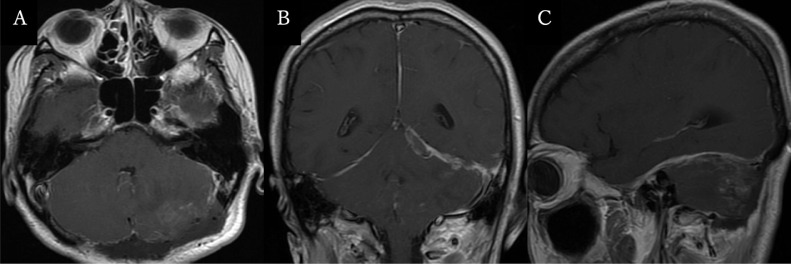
Postoperative magnetic resonance images, axial (A), coronal (B), and sagittal views (C), showing the tumor and hematoma have been largely removed.
